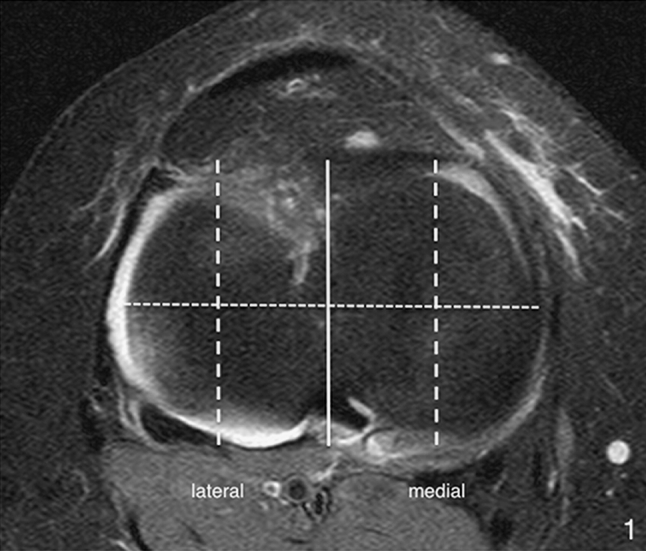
First transverse MR image in craniocaudal direction that shows the entire tibial head. In this transverse image, the corresponding sagittal slices located most closely to the tibial head centre (*solid line*) and in the centre of the medial and lateral tibial plateau (*dashed lines*) were determined. Similarly, the corresponding coronal section located most closely to the tibial head centre (*dotted line*) was established
